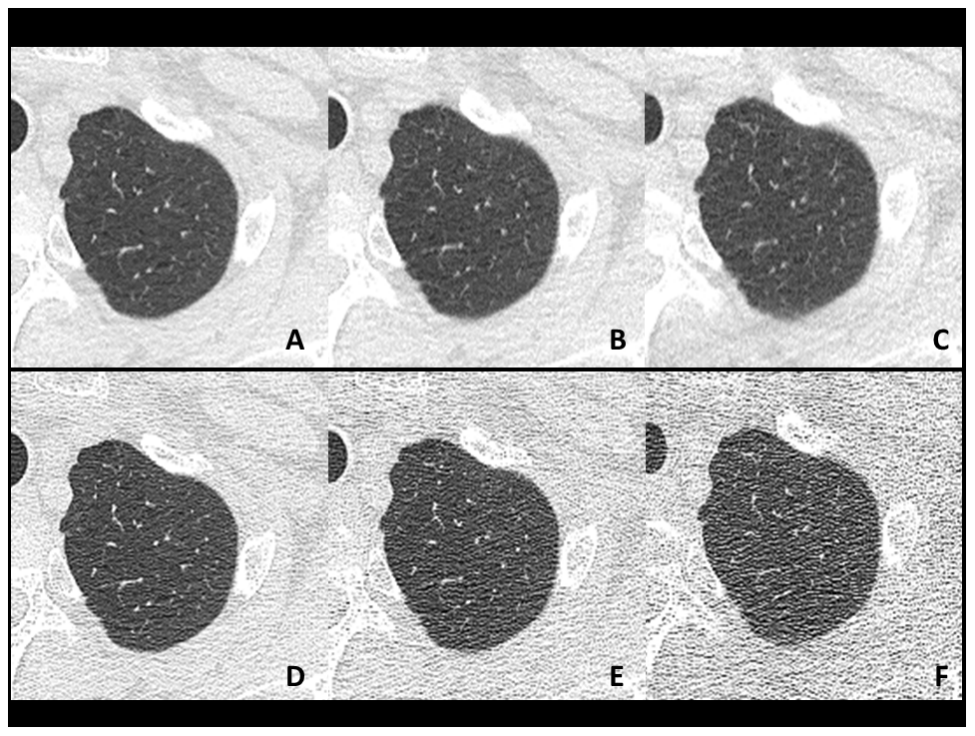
Axial plain chest CT images at the upper lung zone (60-year-old male weighing 76 kg). These images were created from scan data at 240 mA (A, D), 120 mA (B, E) and 60 mA (C, F). The three upper images (A–C) were reconstructed using AIDR3D and the three lower images (D–F) were reconstructed using a conventional reconstruction mode (Boost3D). Each image pair at the same tube current was created from single row data. Image noise was obviously reduced on images with AIDR3D, particularly at lower tube currents.
